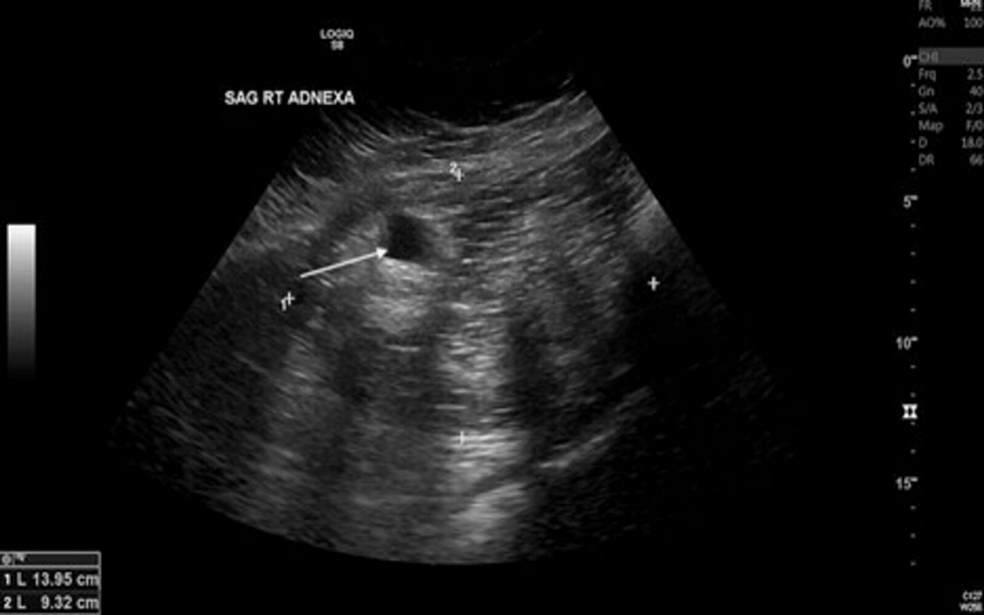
Pelvic ultrasound Arrow denotes ovarian mass.
